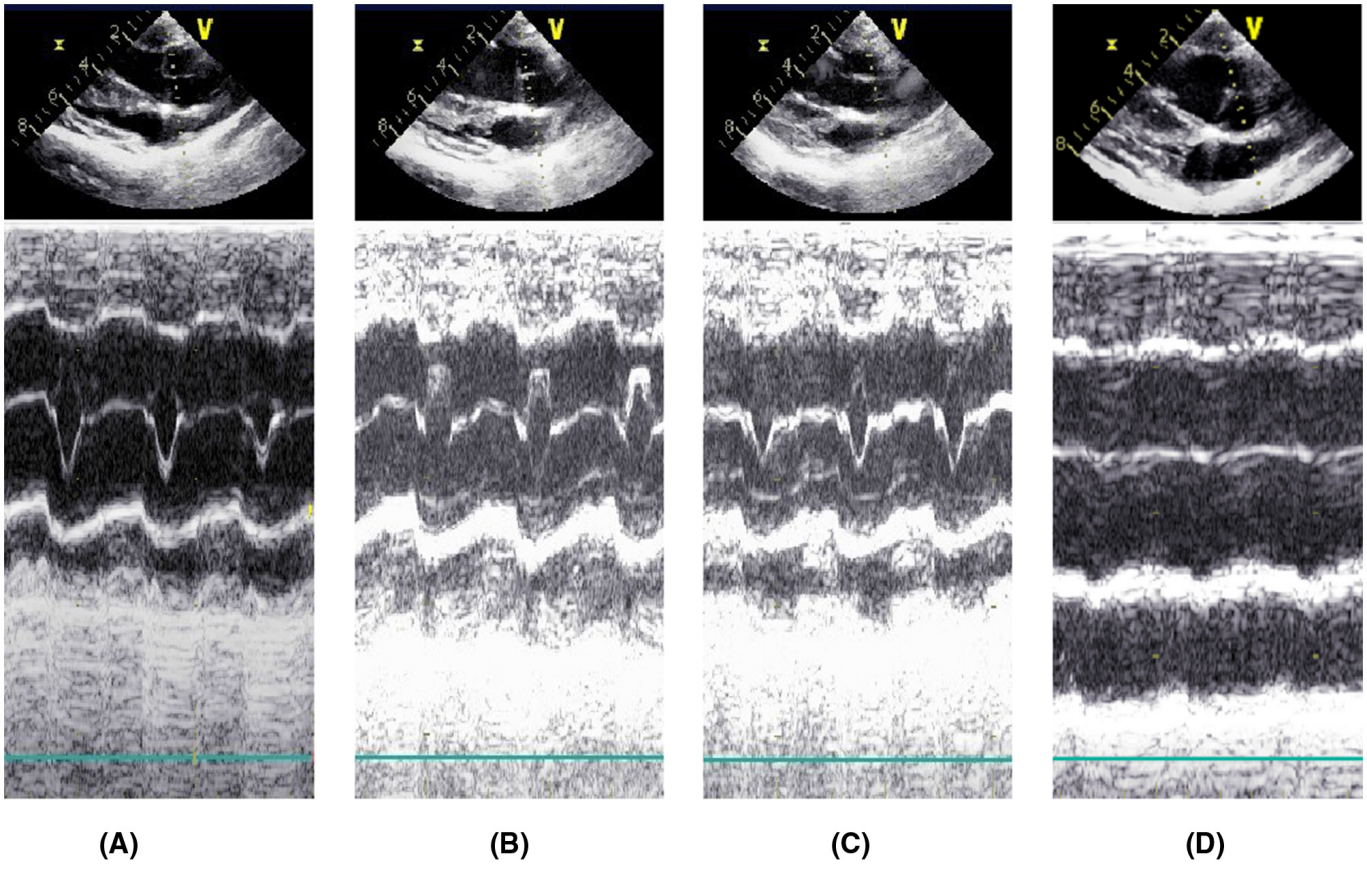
M‐mode echocardiography of the functional status of the aortic valve at a constant HR of 70 beats/min. All ultrasound examinations showed no signs of aortic regurgitation. Aortic valve leaflets open/close 1:1 in A–C. complete closure of the aortic valve leaflets in D. (A) 2100 rpm pump speed, AVO; (B) 2300 rpm pump speed, AVO; (C) 2350 rpm pump speed, AVO; (D) 2400 rpm pump speed, AVC
